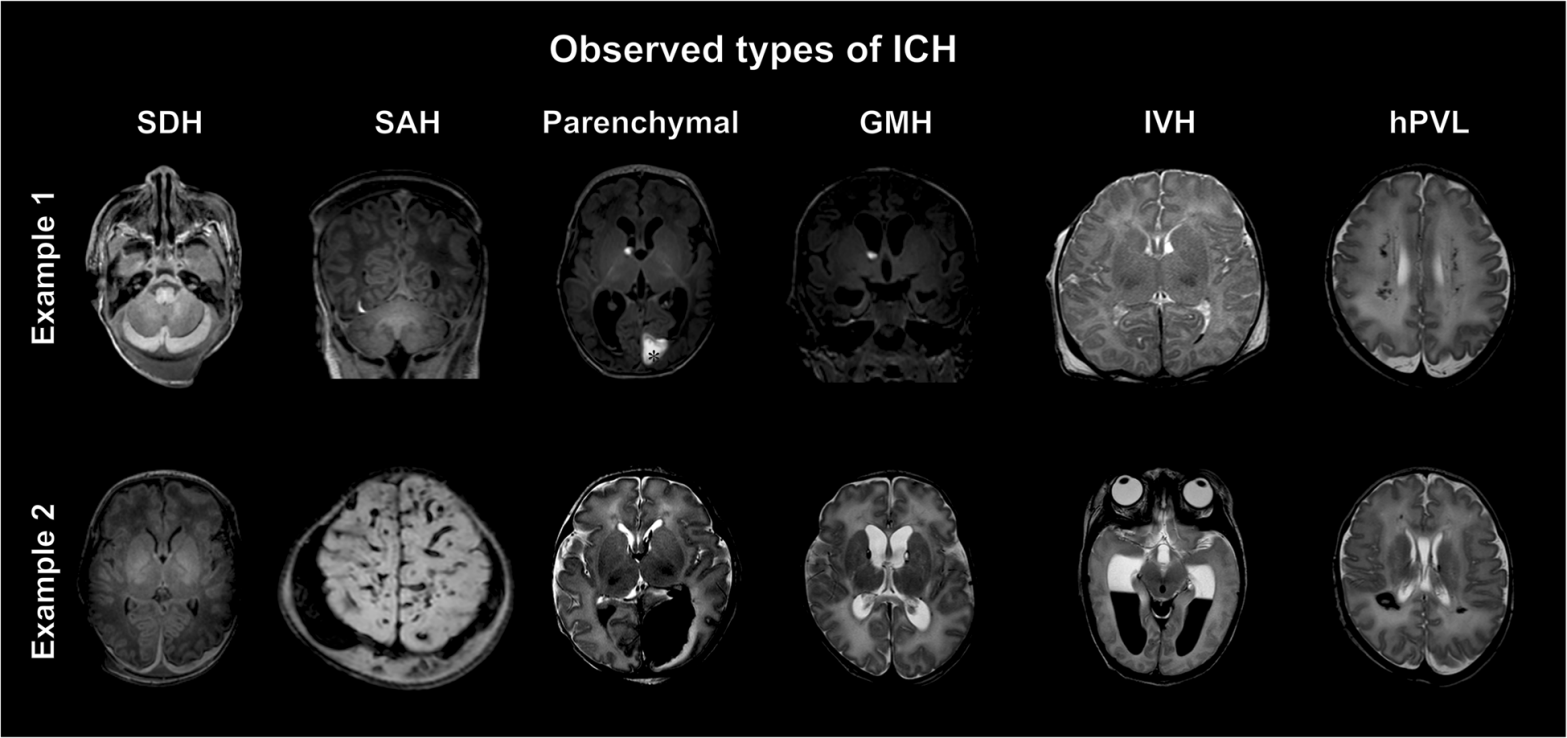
Observed types of ICH. MR images show two sets of examples for intracranial hemorrhages of different types, locations and sizes observed in asphyxiated infants. SDH: subdural hemorrhage, SAH: subarachnoid hemorrhage, GMH: germinal matrix hemorrhage, IVH: intraventricular hemorrhage, hPVL: hemorrhagic periventricular leukomalacia
